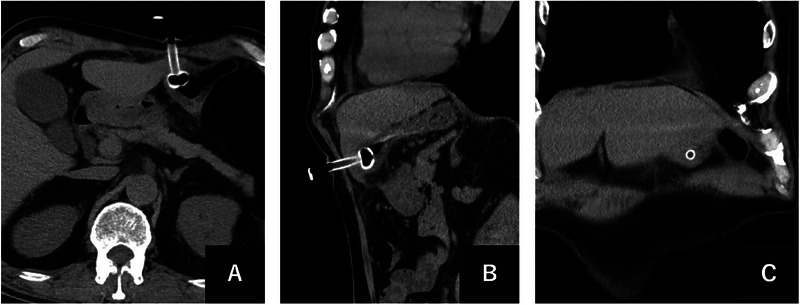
Computed tomography imaging on postoperative day 2 demonstrating transhepatic percutaneous endoscopic gastrostomy tube placement. (A) Axial computed tomography (CT) image showing the 24‐Fr gastrostomy tube penetrating through the thin parenchyma of the hepatic lateral segment before entering the stomach. (B) Sagittal reformatted CT image clearly demonstrating the transhepatic course of the gastrostomy catheter from the abdominal wall through the thin hepatic parenchyma into the gastric lumen. (C) Coronal reformatted CT image showing the gastrostomy tube surrounded by hepatic parenchyma, providing clear visualization of the transhepatic penetration and confirming the inadvertent placement through the hepatic lateral segment.
